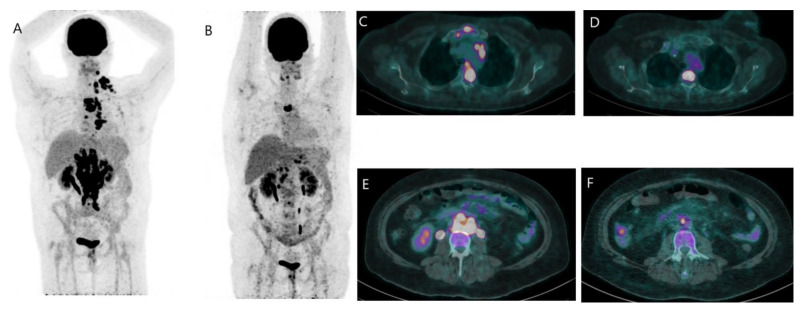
FDG PET/CT in evaluation of treatment response. A 47-year-old woman with metastatic HER2+ BR was treated by paclitaxel and pertuzumab and was referred for evaluation of treatment response. The PET/CT MIP images (A,B) show a significant partial metabolic response at 3 months of interval. Images (C,D) (fusion image, trans-axial view) show the regression of mediastinal adenopathy and sternum lesion and also the persistence of a bone lesion of T4. Images (E,F) (fusion image, trans-axial view) show the important regression of retroperitoneal lymph node involvement.
